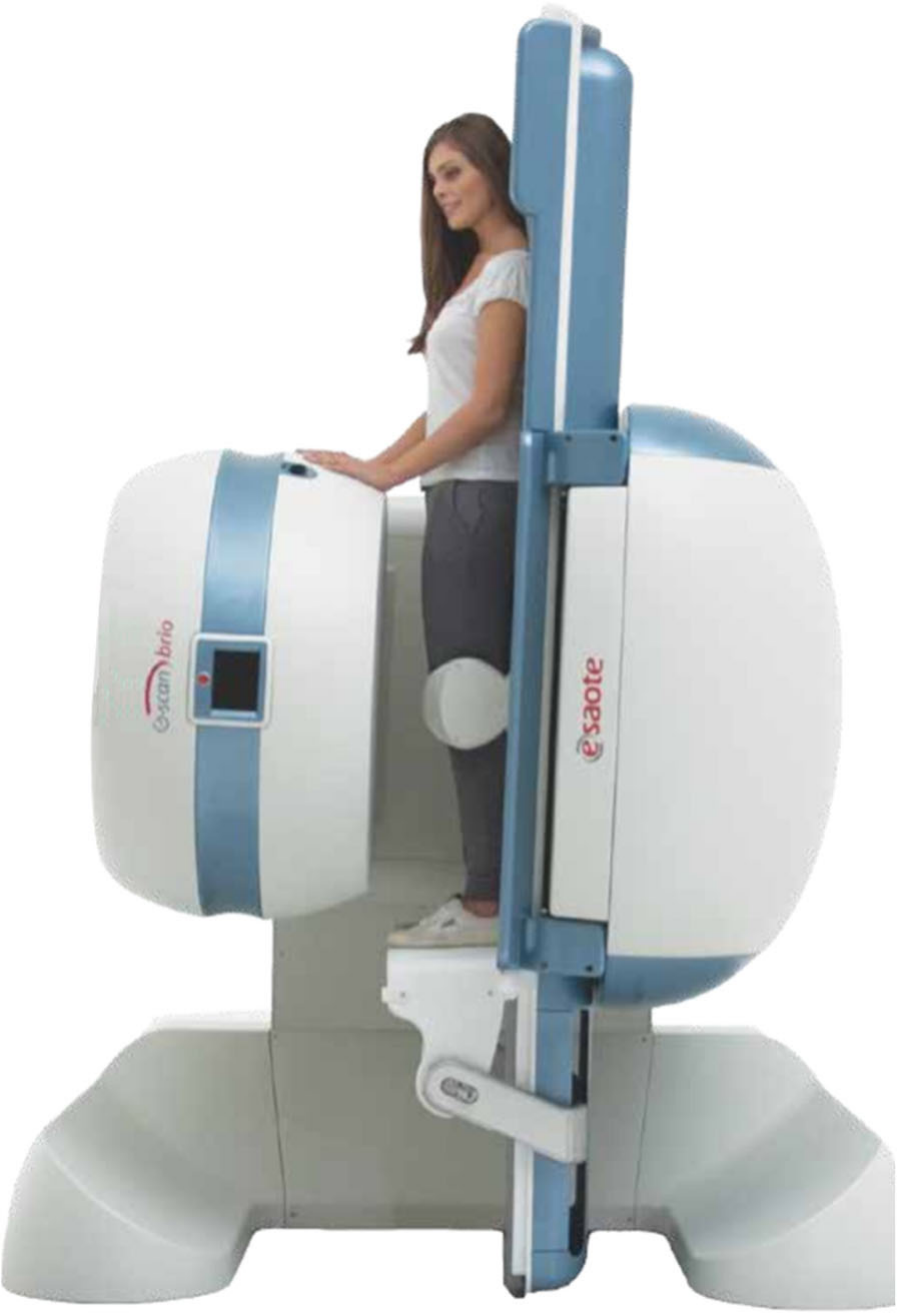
Scanning position in weight-bearing condition in a low-field MRI-scanner. Adapted with permission from Esaote (esaote.com)
